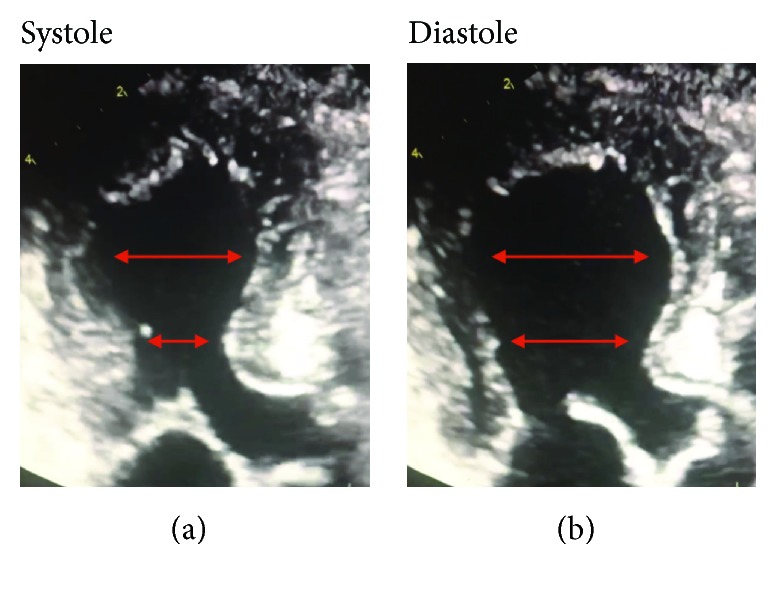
Apical three chamber echocardiogram demonstrating left ventricular apical ballooning and basal hyperkinesis consistent with takotsubo syndrome.
